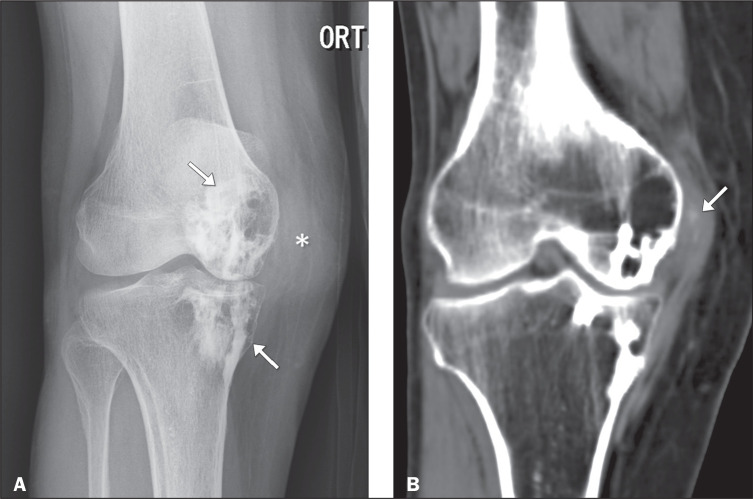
Anteroposterior X-ray of the knee (A) of a 75-year-old patient with a
